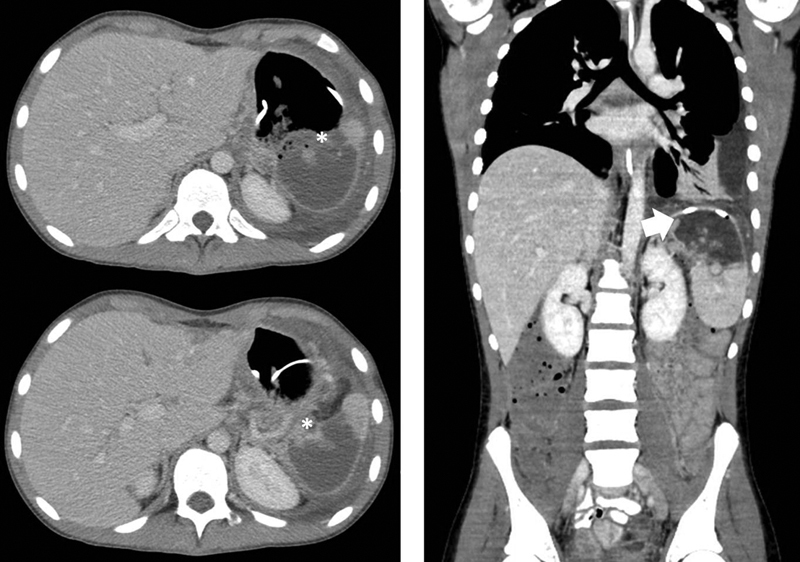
CT scan images demonstrating gastrosplenic fistula. (*) shows fistulous communication between stomach and spleen. Arrowhead represents coiled feeding tube within the capsule of the spleen. CT, computed tomography.
